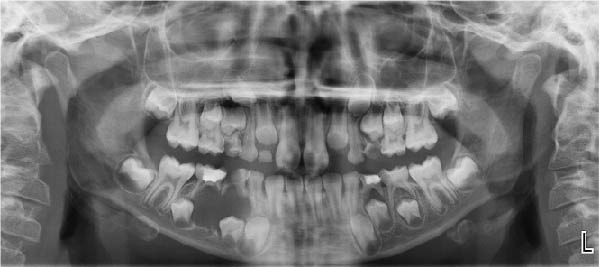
Pretreatment panoramic radiograph showing apical displacement of teeth #44 and #45 toward the inferior border of the mandible.
